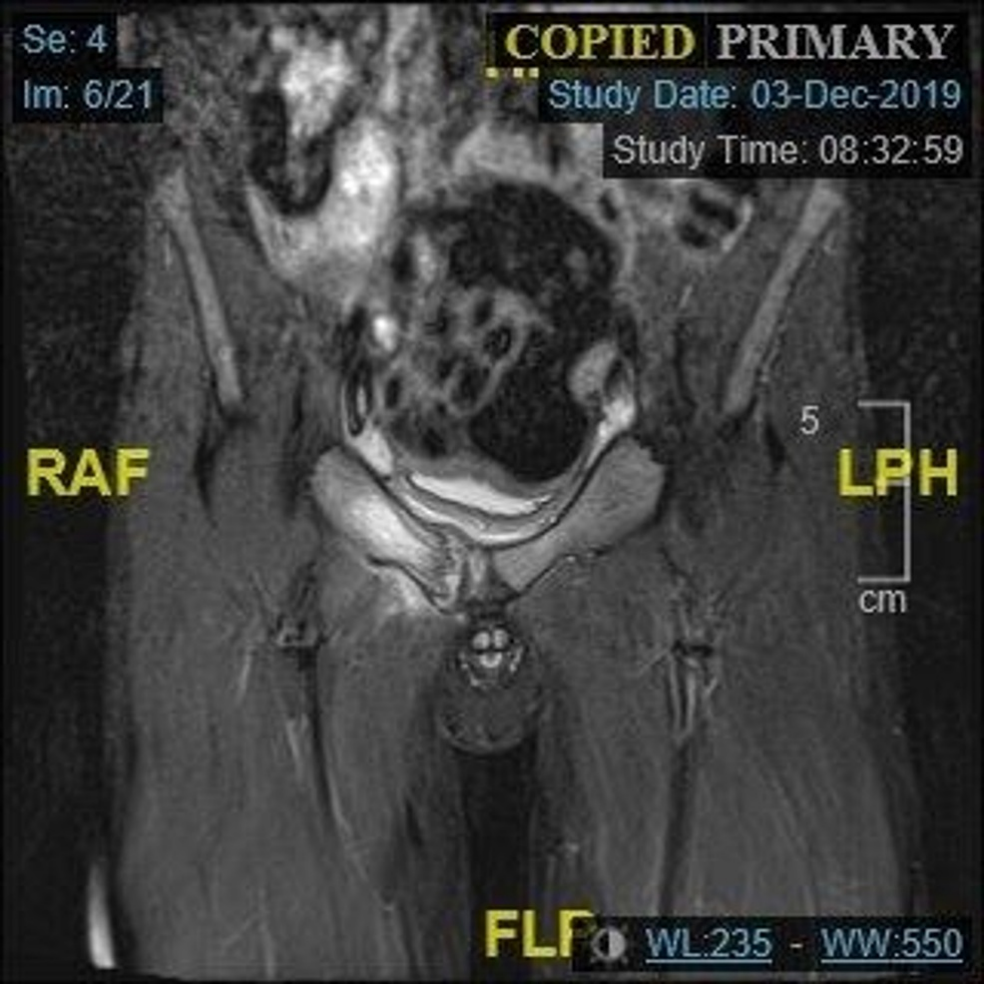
Coronal sections of T2-weighted MRI demonstrating marked oedema in the right parasymphyseal region, likely in keeping with a further area of stress reaction or stress fracture.
